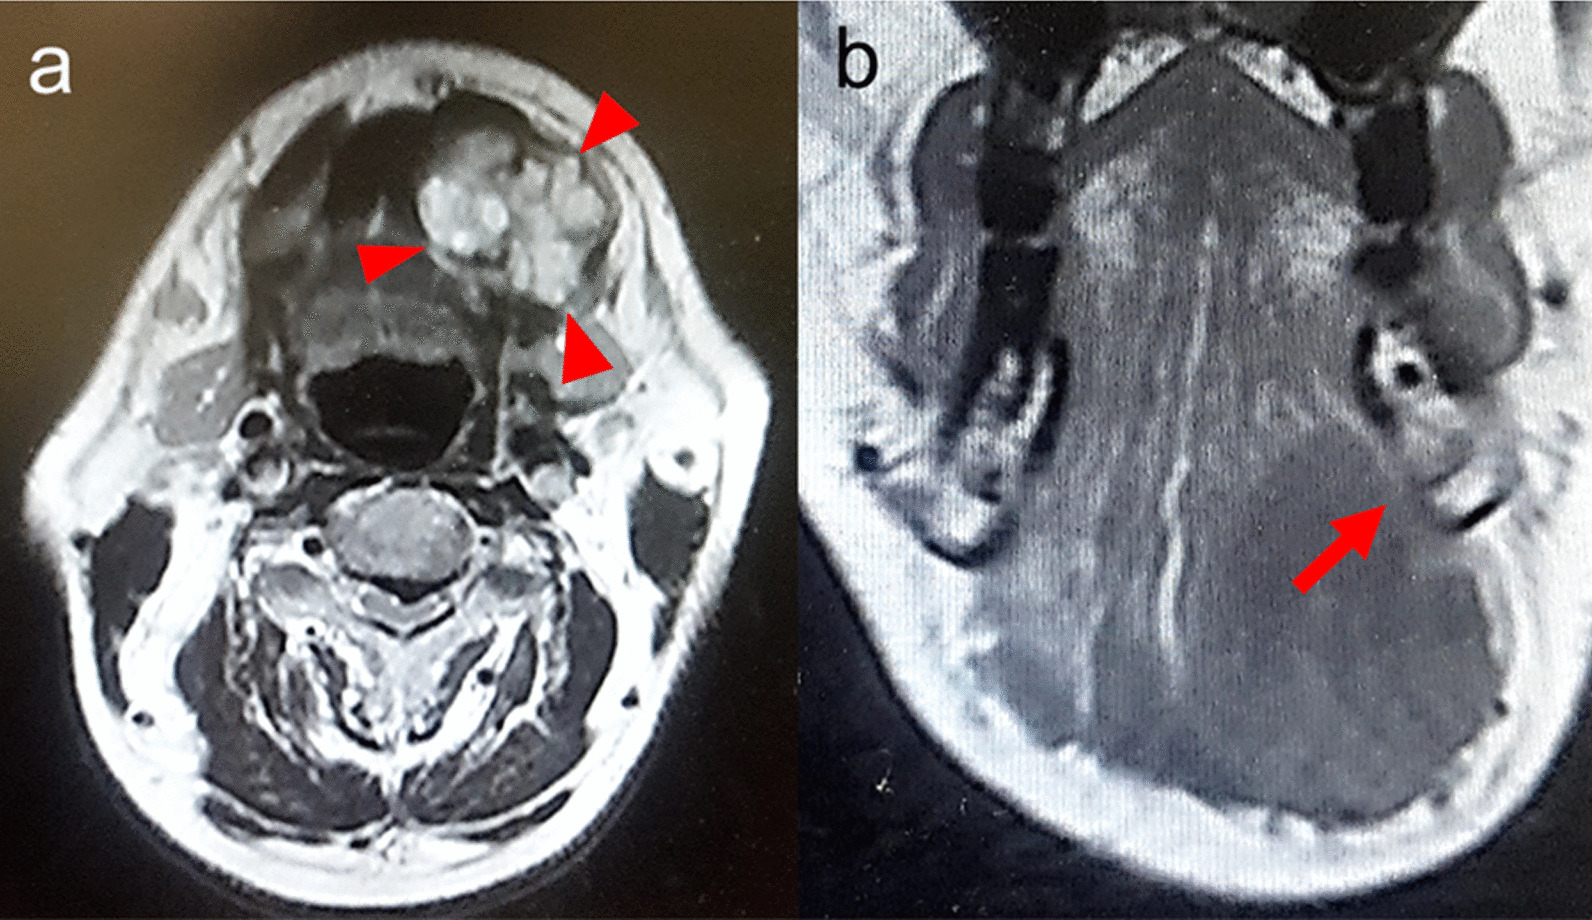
Magnetic resonance imaging. a Magnetic resonance imaging (T1-weighted image) revealed a well-circumscribed lobulated mass on the left side of the floor of the oral mouth (arrowhead). b A frontal section (T2-weighted image) shows an expanded mass adjacent to the mandible without connection to the gingival mucosa or jawbone (arrow)
